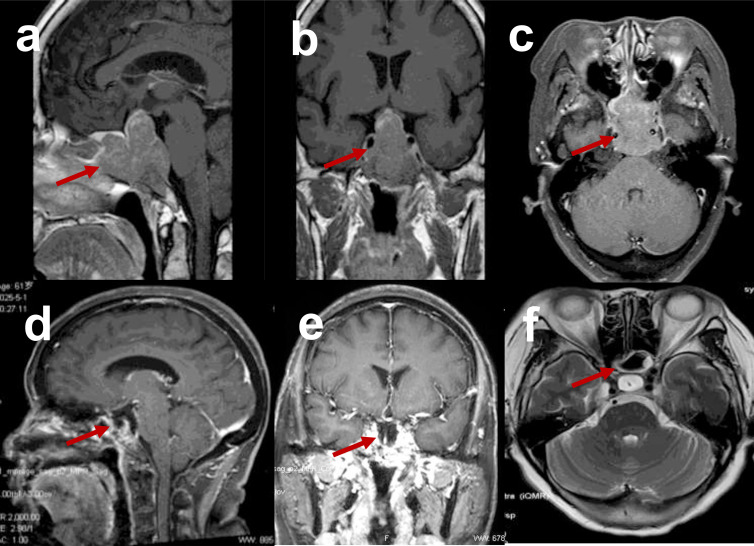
Comparison of preoperative and postoperative contrast-enhanced MRI scans of the brain. Preoperative MRI demonstrated a well-defined, irregular mass within the sellar/suprasellar regions and sphenoid sinus. T1-weighted images showed a homogeneous isointense mass with internal nodular hyperintense foci. The lesion was primarily located within the sellar region, obscuring the pituitary gland and pituitary stalk. It exhibited a drill-like growth pattern, accompanied by osteolytic destruction of the sphenoid sinus wall and skull base. The optic chiasm was displaced superiorly due to compression from the mass (a–c). Seven months after surgery and chemotherapy, brain MRI revealed no tumor (d–f).
